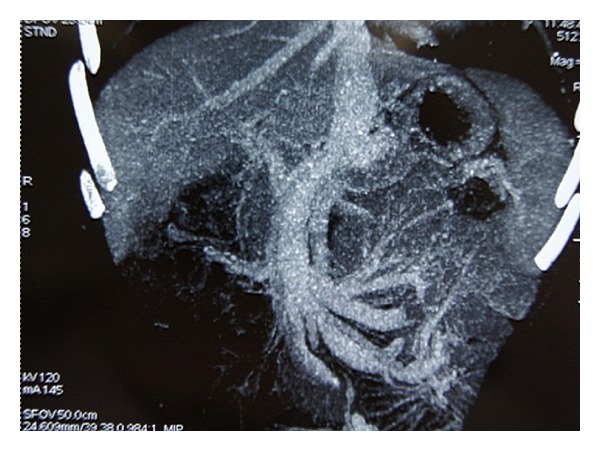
CTA reveals that intestinal and splenic venous blood bypassed the liver and drains into the inferior vena cava.
